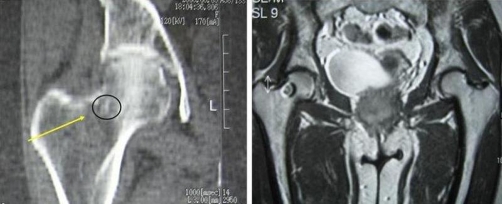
The CT representation of the lesion 3 months after RF ablation (left). The MRI representation of the lesion before RF ablation (right).
